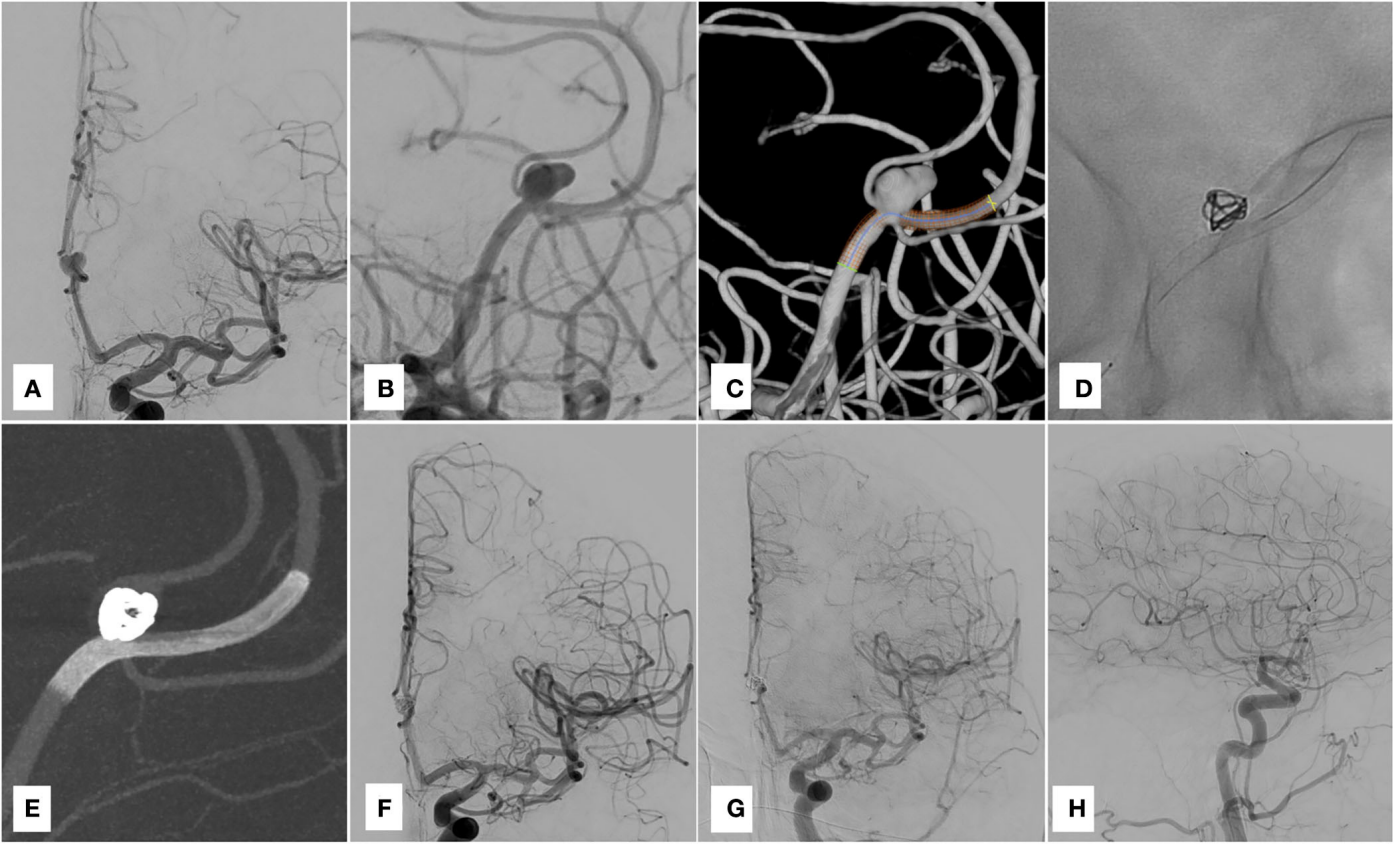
Case 2. (A–C) Pre-treatment DSA and 3D reconstruction. (D) Derivo 2 deployed. (E,F) Post-treatment run and Vaso-CT. (G,H) Six-month-DSA follow-up.
